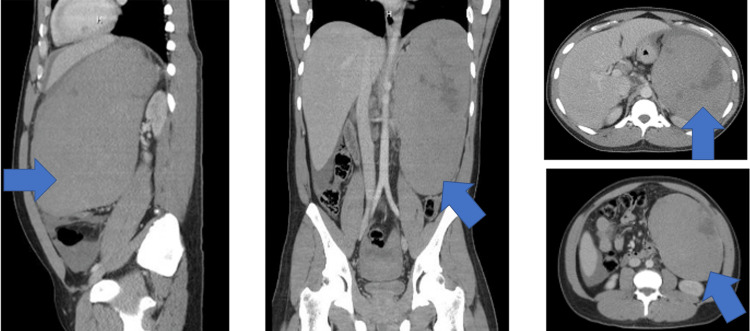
Computed tomography: lateral, coronal, and cross-sectional views of the abdomen with findings concerning massive splenomegaly measuring approximately 25 cm (blue arrow). Irregular hypoattenuating areas within the inferolateral and superolateral aspects of the spleen with small to moderate volume hemoperitoneum.
